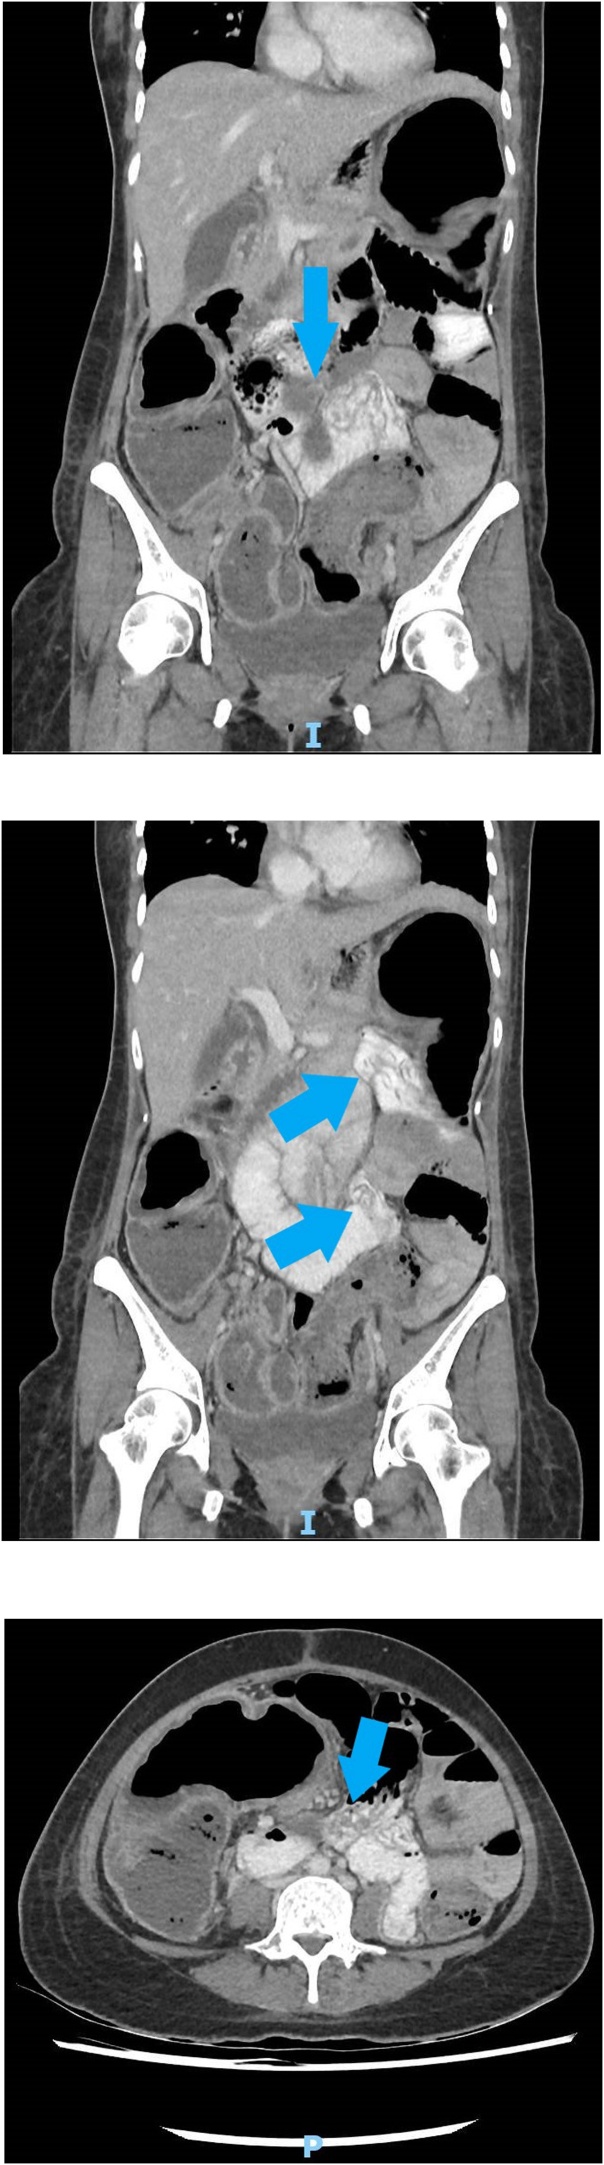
CT scan of possible small bowel worm infestation.
